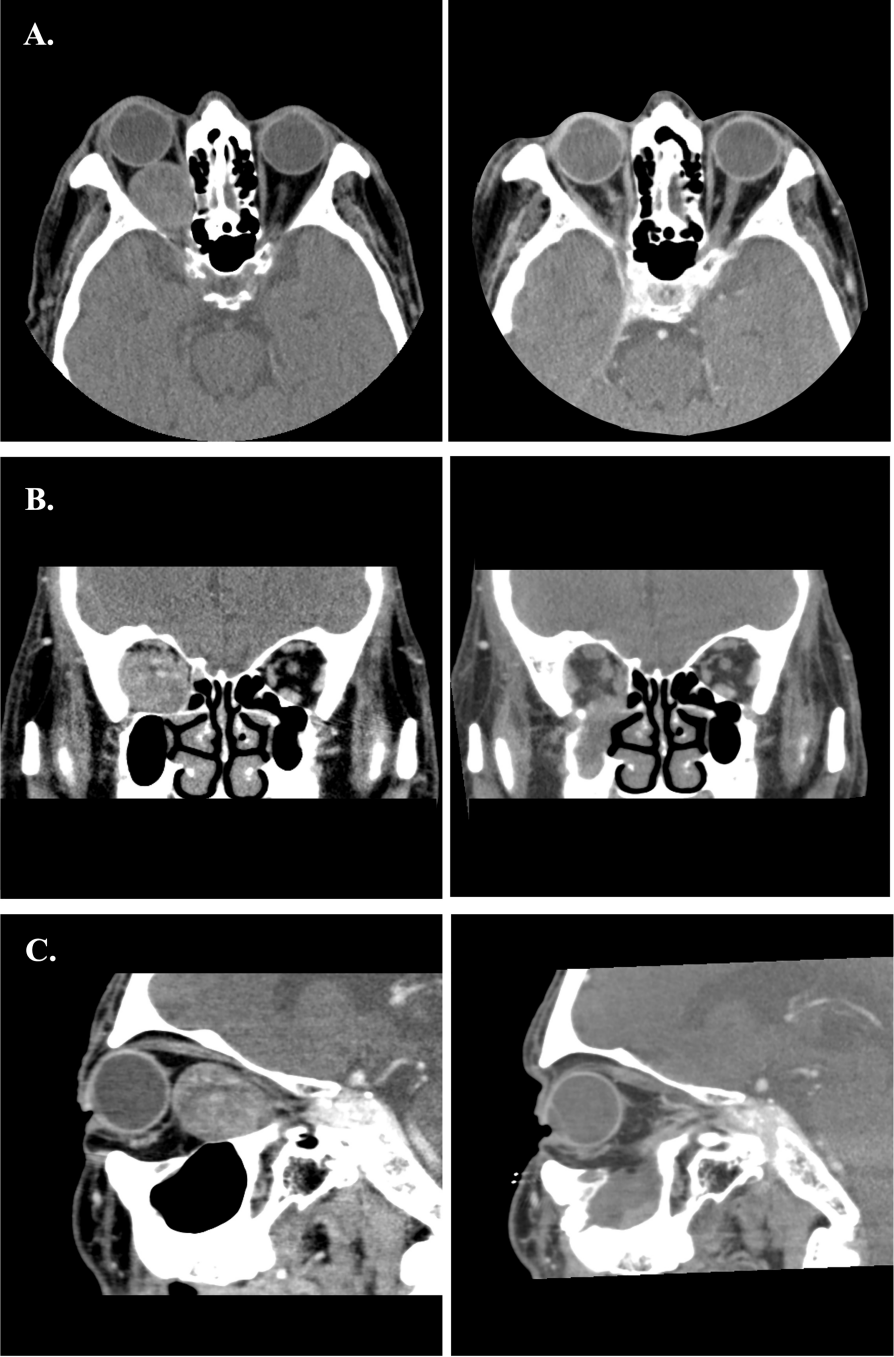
(A–C) Preoperative and postoperative images of Case 1. The left-side axial, coronal and sagittal images were the preoperative CT scans. The right-side postoperative CT images showed normal extraocular muscles position and well restored orbital volume.
